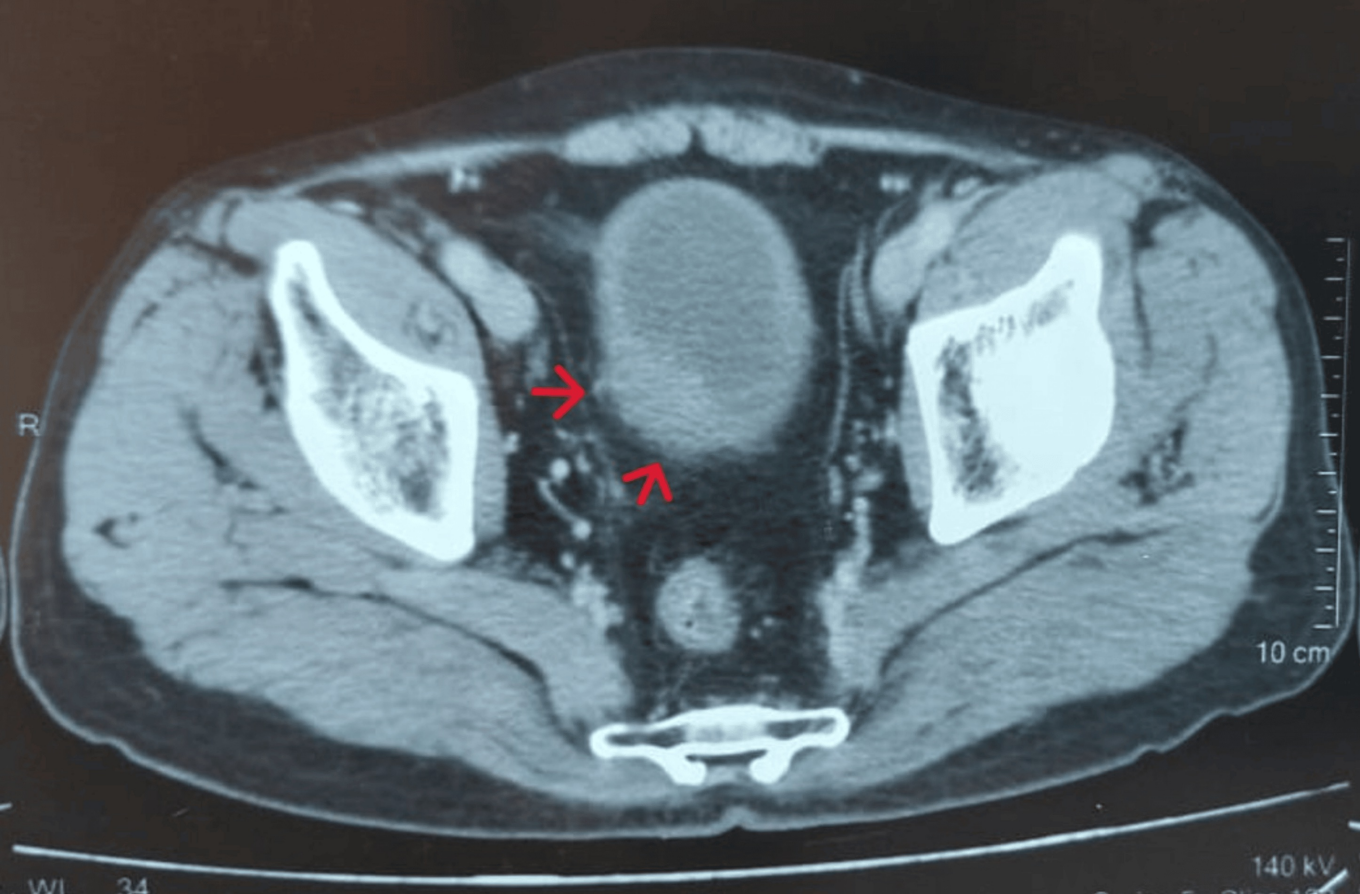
The CT scan showed a circumferential tumoral lesion of the bladder with poorly defined margins and infiltration of peri vesical fat (red arrows).
